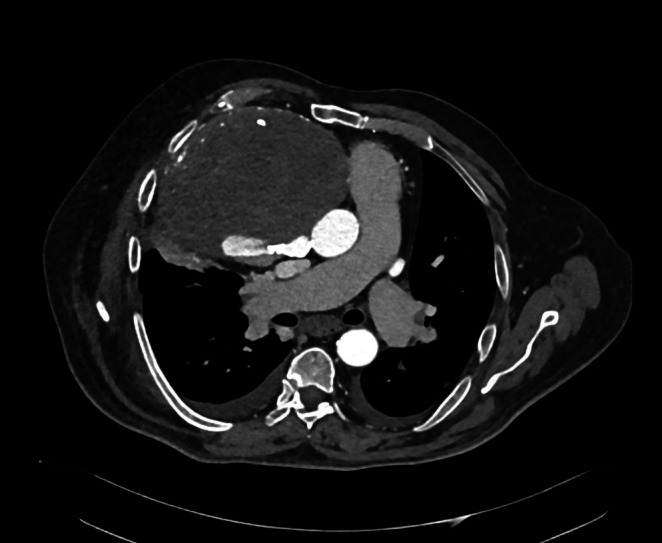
CTA, axial plane, arterial phase. The communication with RCA proximal tract can be noticed.
